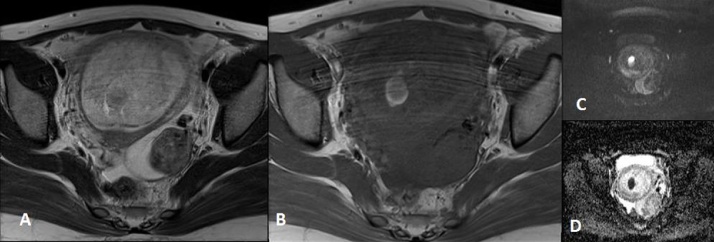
Molar pregnancy in a 24-year-old woman. (A) Perpendicular to the endometrial line T2-weighted MR image of the pelvis showing a snowstorm appearance. (B) Axial T1-weighted MR image showing focal hypersignal for a round and crescent intratumoral hemorrhage, (C) DWI with a b value of 800 showing the same hemorrhage as a bright focus, and (D) an ADC map showing the same hemorrhage as a signal void focus.
